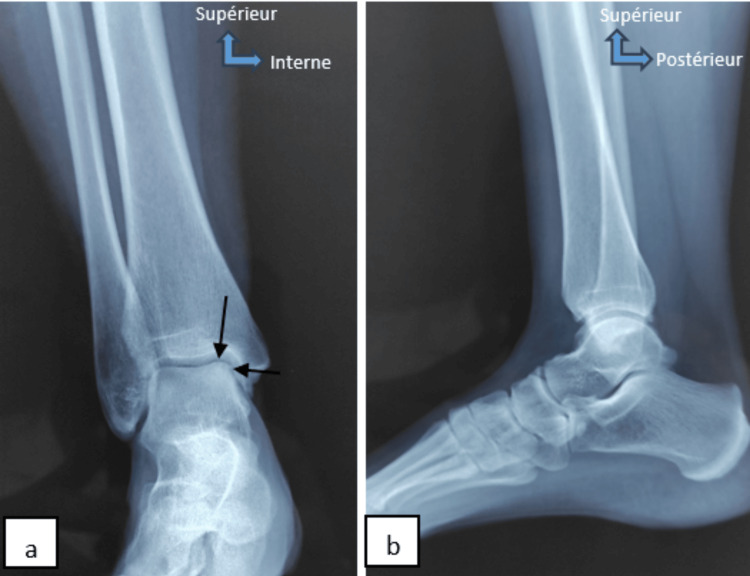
X-ray of the right ankle of case 2 (face (a), profile(b)) shows osteochondral lesions of the anteromedial part of the talus dome (black arrows).
